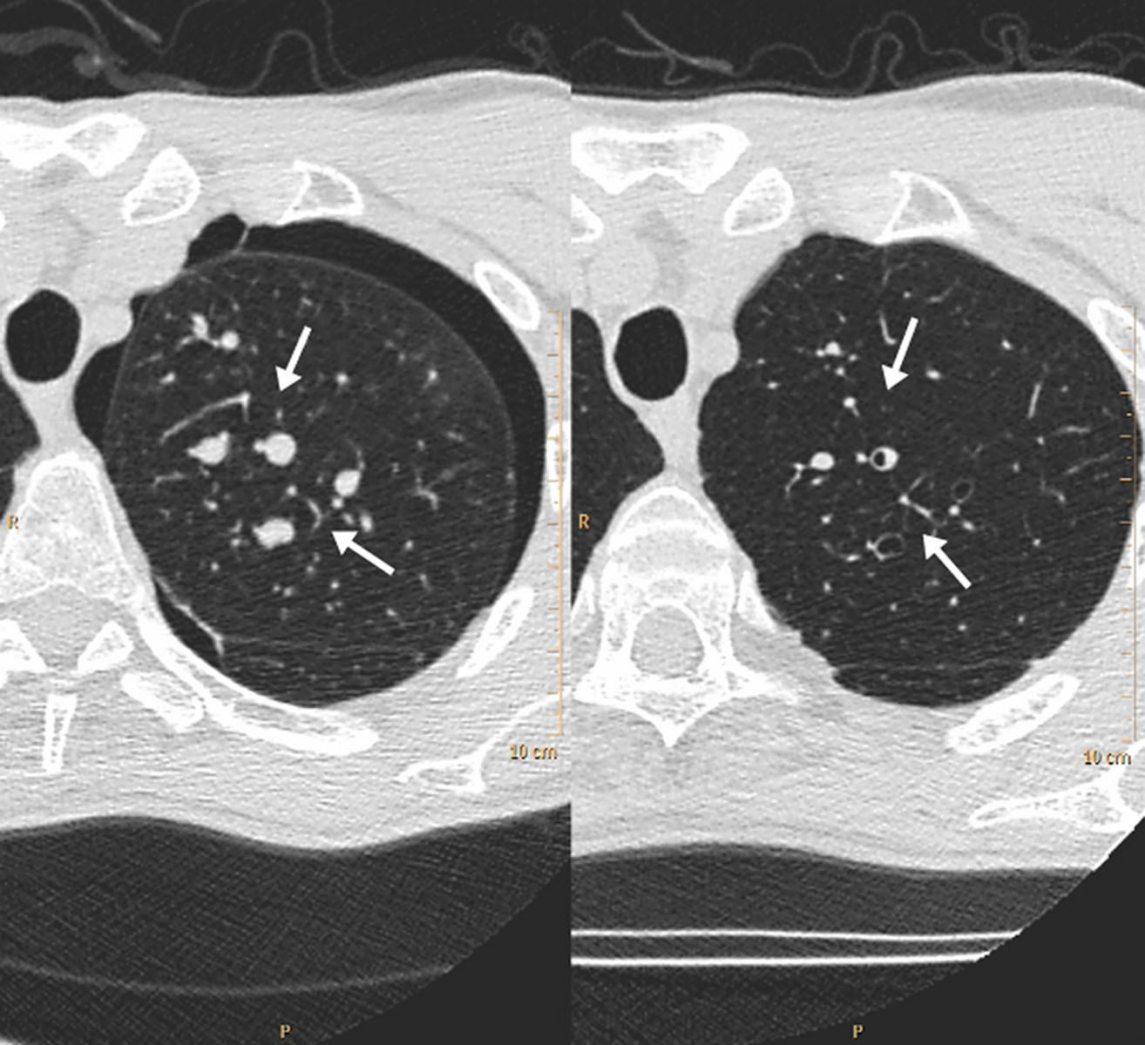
CT image at the same level before and following treatment at six weeks. Arrows annotate the fluid filled dilated bronchi which demonstrate improvement following treatment.
